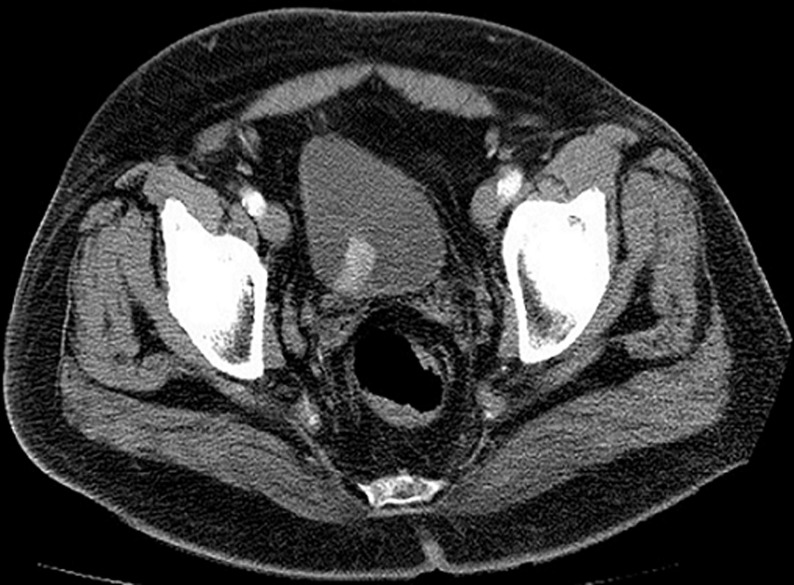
CT scan showing bladder lesion
